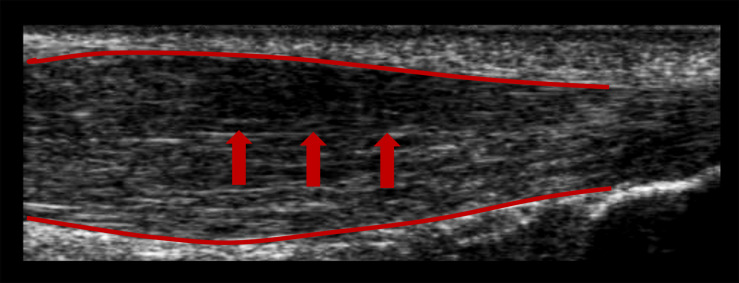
B-mode ultrasound image showing a hypoechogenic area (arrows) and spindle-shaped thickening (red lines) of a symptomatic Achilles tendon.
